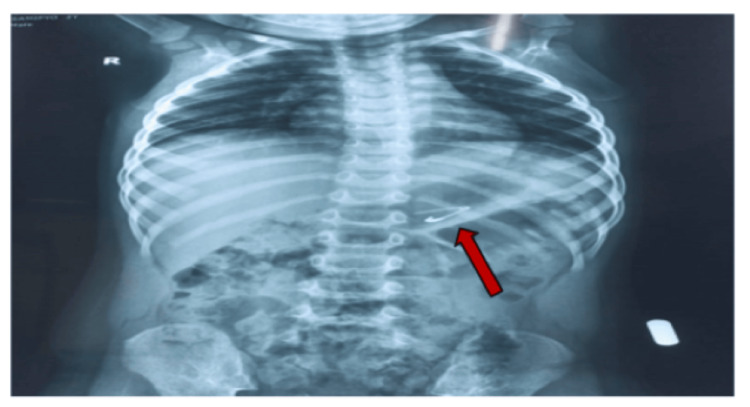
An X-ray of the abdomen showing a foreign body, an open safety pin, in the abdomen.
